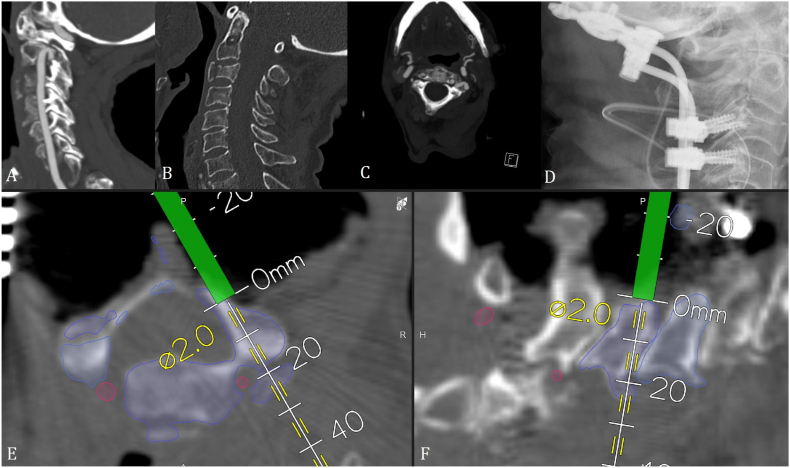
Patient No. 33. Preoperative sagittal A. CT angiography and B. CT of the cervical spine showing a Hangman's fracture with displacement and high-riding vertebral artery on the right side, as well as a C3 fracture. C. Axial CT angiography of the Hangman fracture. D. Postoperative X-ray after occipito-cervical stabilization. Intraoperative screenshots with drill guide for the implantation of a lateral mass screw in E. axial and F. sagittal view.
